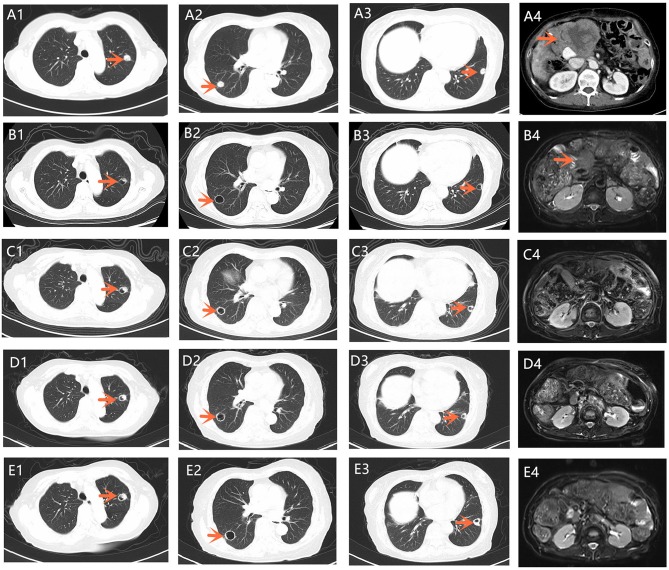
(A1–A4) A chest CT scan showed multiple metastases of both lungs. Total abdominal CT scan revealed a 7.8 × 6.9 cm mass located in the original gallbladder area, accompanied by abdominal and retroperitoneal lymph node metastasis (September 1, 2018); (B1–B4) A chest CT scan and T2-weighted abdominal MRI on November 16, 2018. The density of pulmonary metastases had considerably decreased with cavity formation compared with that in (A1–A3). Tumor shrinkage was confirmed 2 months after apatinib was orally administered. (C1–C4) A chest CT scan and T2-weighted abdominal MRI on February 20, 2019. The mass located in the original gallbladder area reduced to the point of vanishing completely. (D1–D4) A chest CT scan and T2-weighted abdominal MRI on June 12, 2019. (E1–E4) A chest CT scan and T2-weighted abdominal MRI on September 16, 2019. CT, computed tomography; MRI, magnetic resonance imaging.
